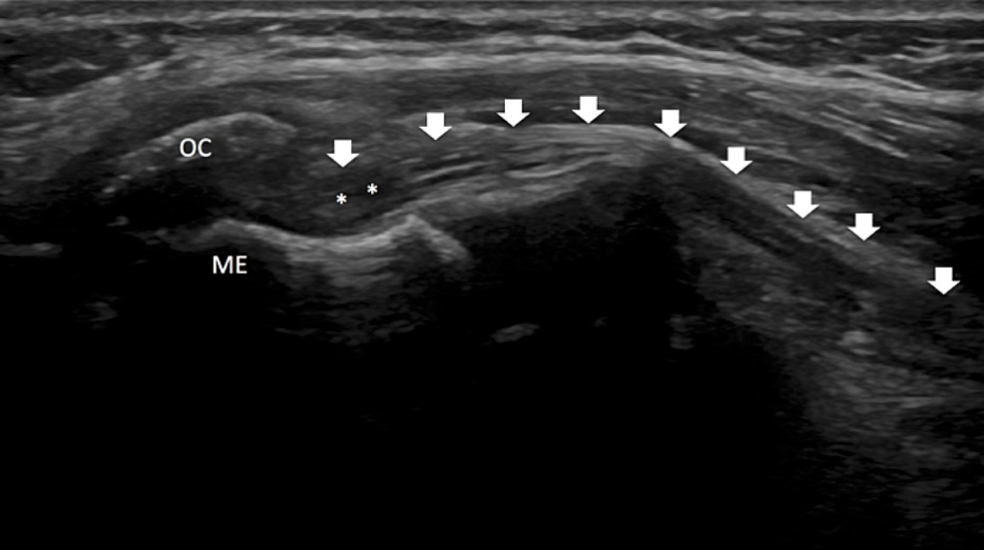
Six-month postoperative ultrasound examination demonstrating the median nerve (arrows) which is seen to deviate deep to the medial ossification centre at the level of the medial epicondyle where it is obscured by acoustic shadowing. There is focal hypoechogenicity of the median nerve just distal to this osseous tunnel consistent with intraneural oedema. The appearances are consistent with intraosseous type 2 median nerve entrapment.
